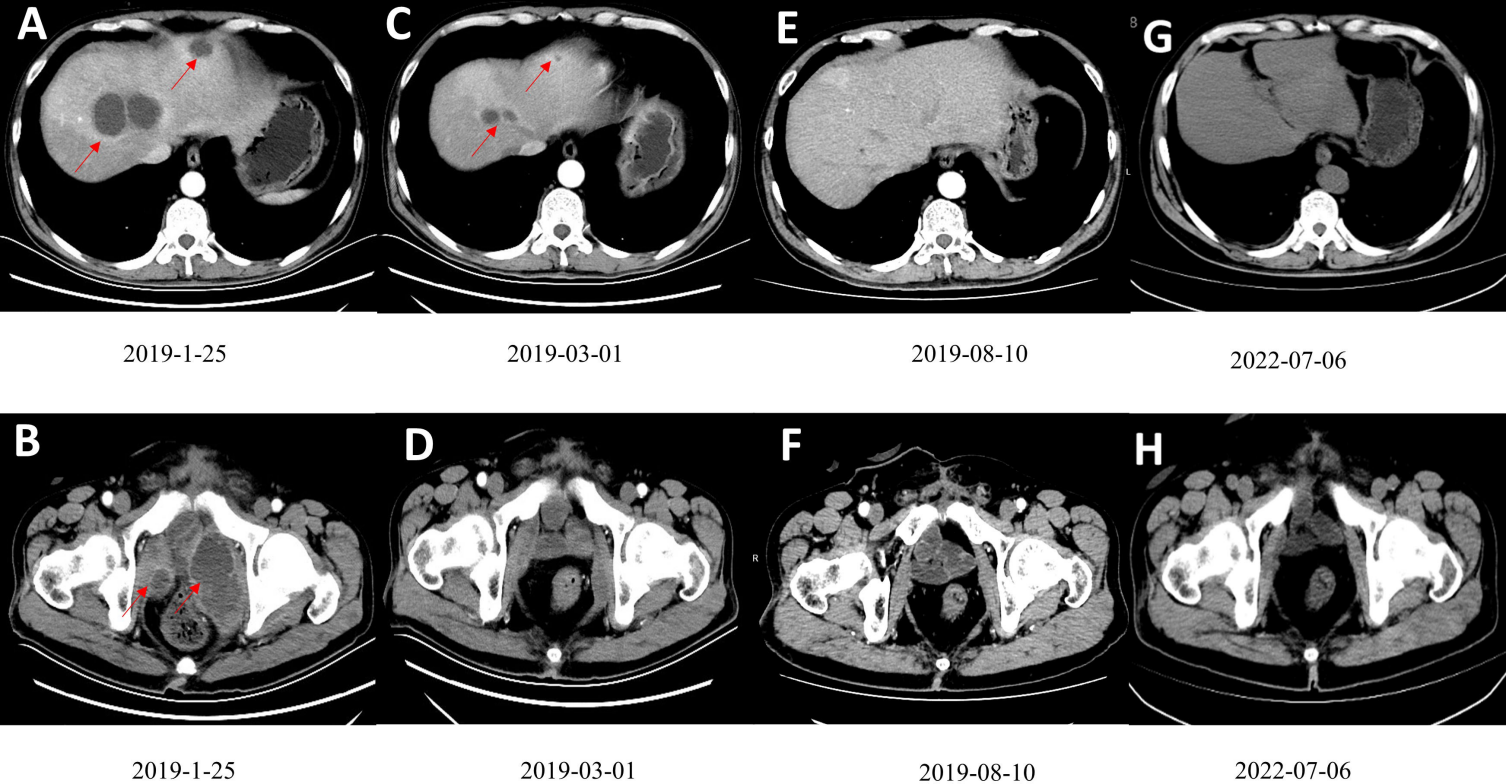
(A, B) Contrast-enhanced CT scan showed multiple low-density tumors of various sizes in the pelvis and liver, and the largest liver metastatic tumor was 4.6cm×3.9cm. (C, D) A contrast-enhanced CT scan after three courses of treatment showed that the tumors in the pelvis and liver had shrunk significantly. (E, F) After ten cycles of treatment, CR was observed. (G, H). The patient had been disease free for 41 months. Figures G and F are the most recent follow-up results.
